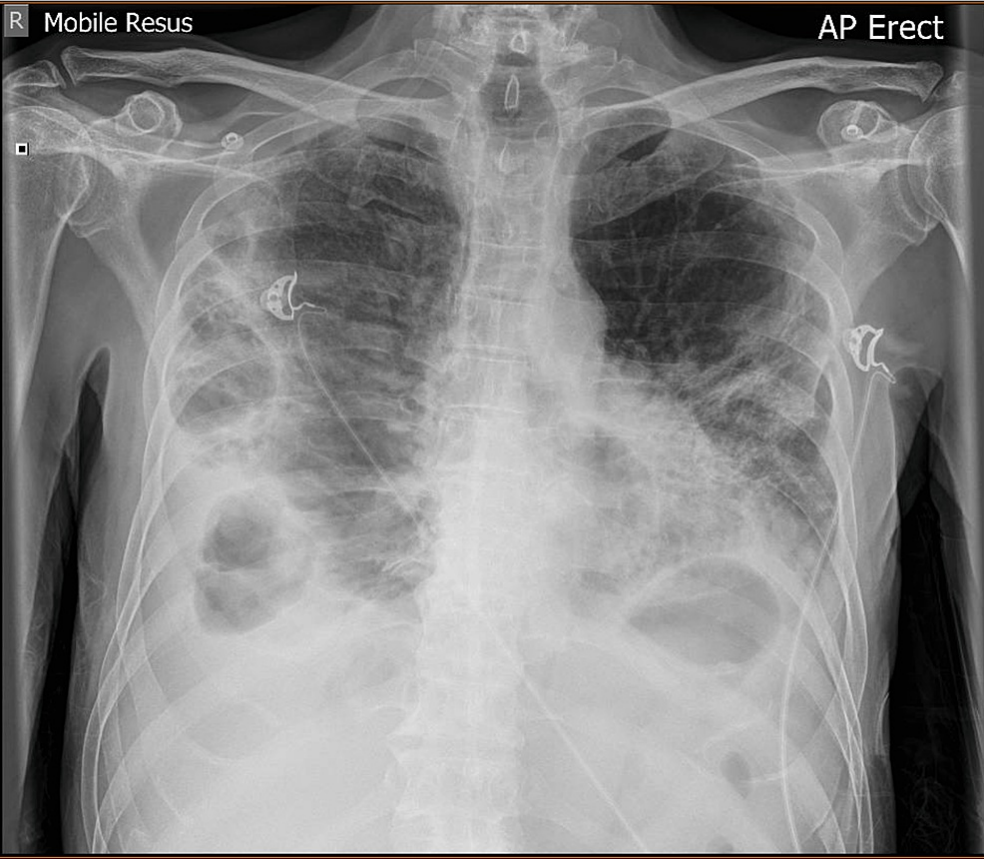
Chest X-ray on day 0 of current admission
